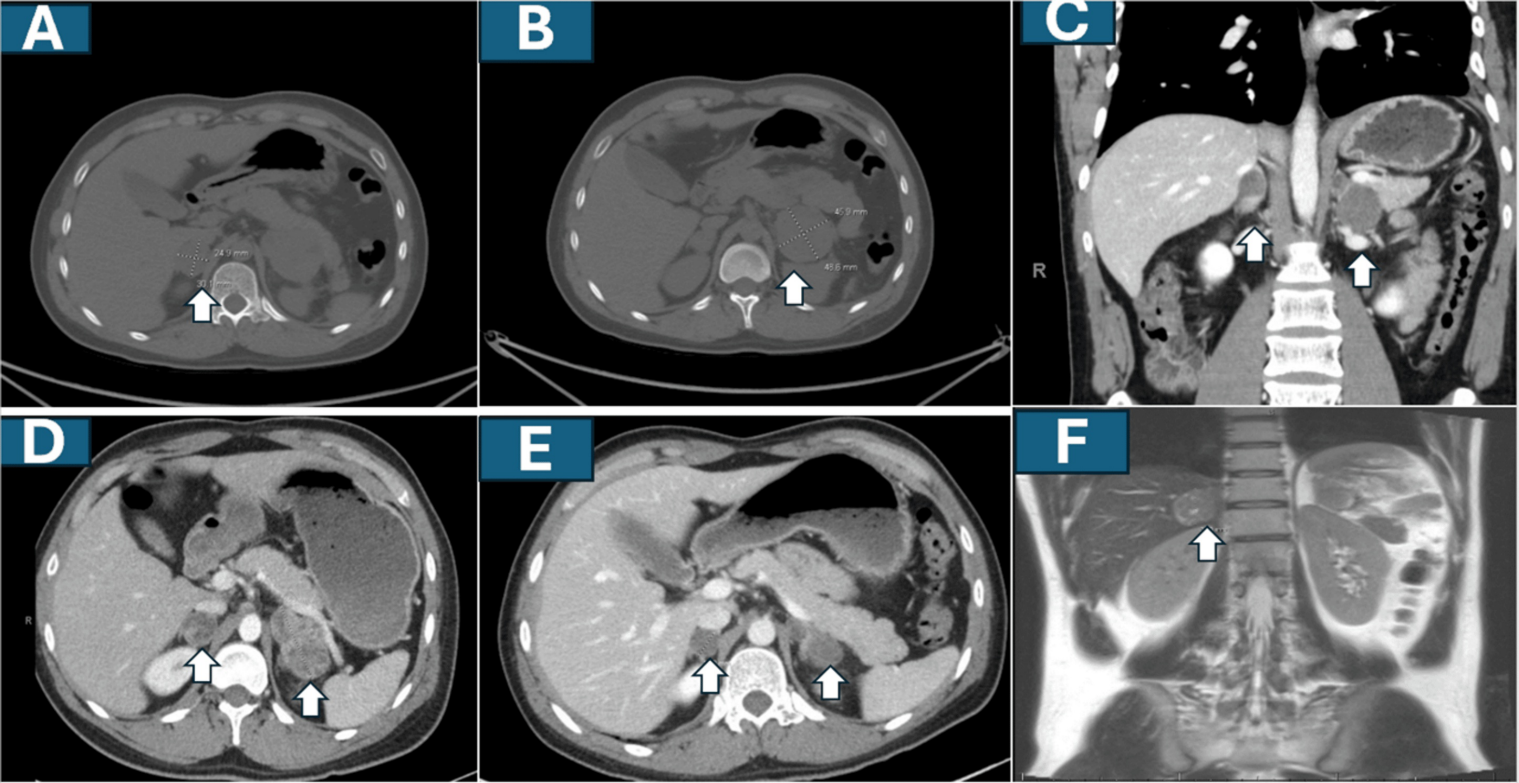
Sequential representative CT (A-E) and MRI (F) images showing patient's disease course after pulmonary metastasectomy A shows an axial CT slice with right adrenal metastasis (white arrow); B shows in another axial view left adrenal metastasis (white arrow); C is a coronal CT view showing bilateral adrenal metastases (white arrows). D and E show axial CT images showing partial response to cabozantinib leading to bilateral oligometastatectomy of adrenals (white arrows). MRI coronal view in F shows recurrence in the right adrenal tumor bed prior to SABR preoperative radiotherapy (white arrow). SABR: Stereotactic ablative body radiotherapy
